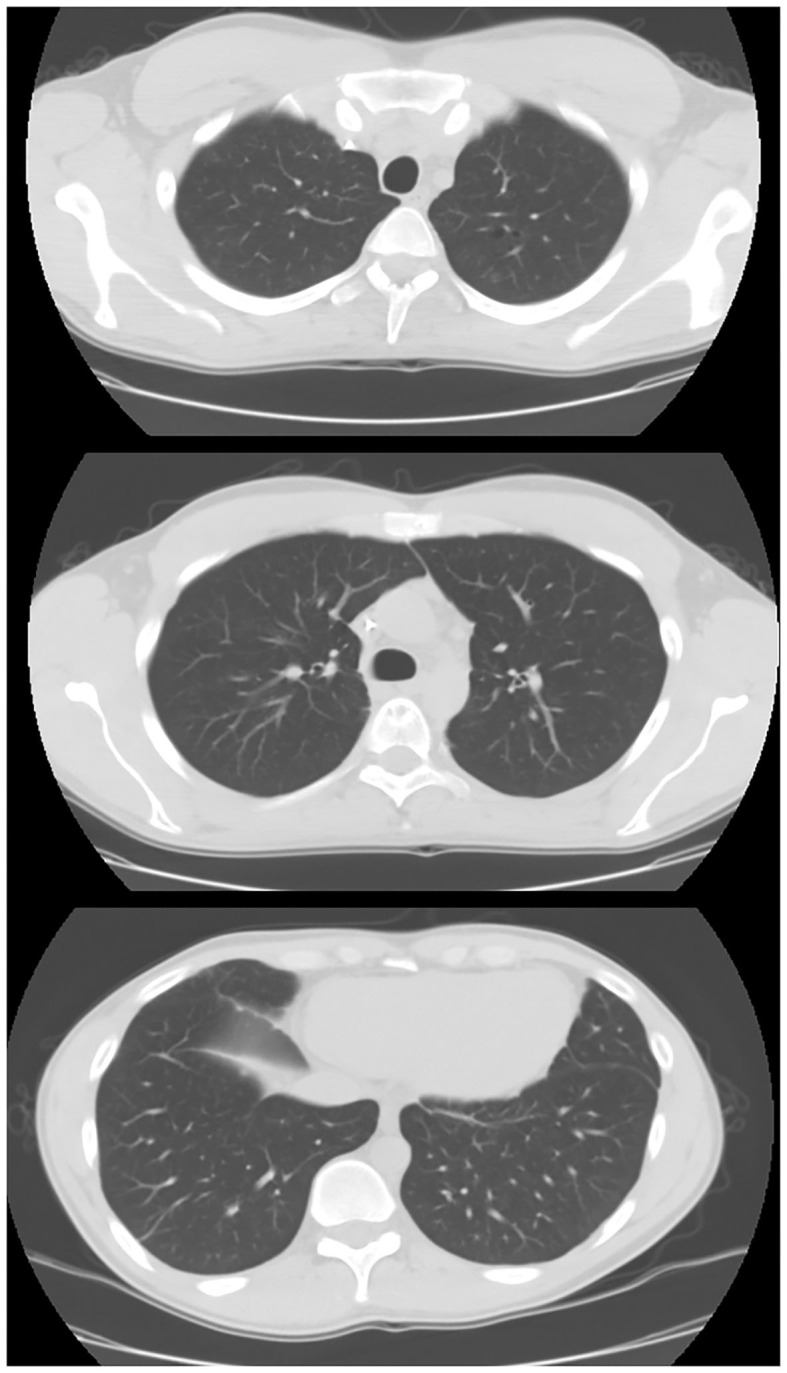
Chest CT in case 1 after chemo- and radiotherapy treatment. After 1 year of treatment, the patient showed a complete remission of the disease.
